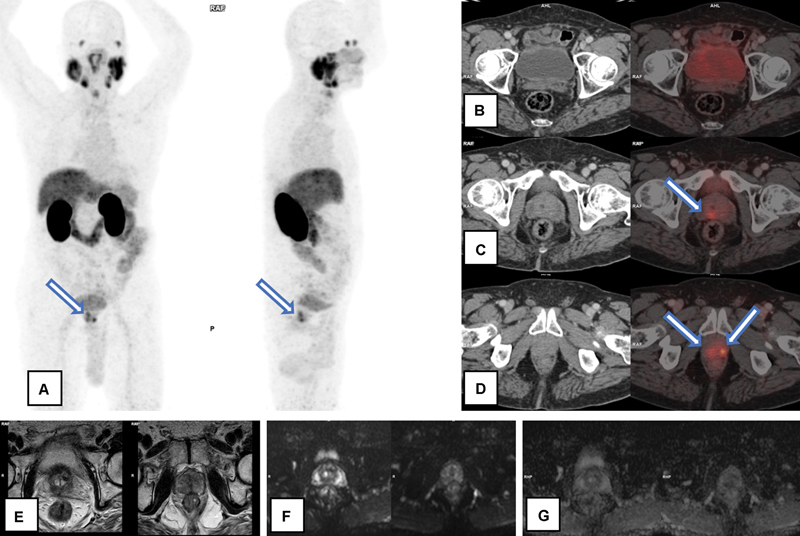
A 66-year-old patient with serum prostate-specific antigen—8.6 ng/mL, adenocarcinoma prostate with Gleason's score 3 + 4- Discordance between gallium-68 prostate-specific membrane antigen positron emission tomography-computed tomography (Ga-68 PSMA PET/CT) and multiparametric magnetic resonance imaging (mpMRI) in primary prostate lesion detection: Ga-68 PSMA PET/CT (
